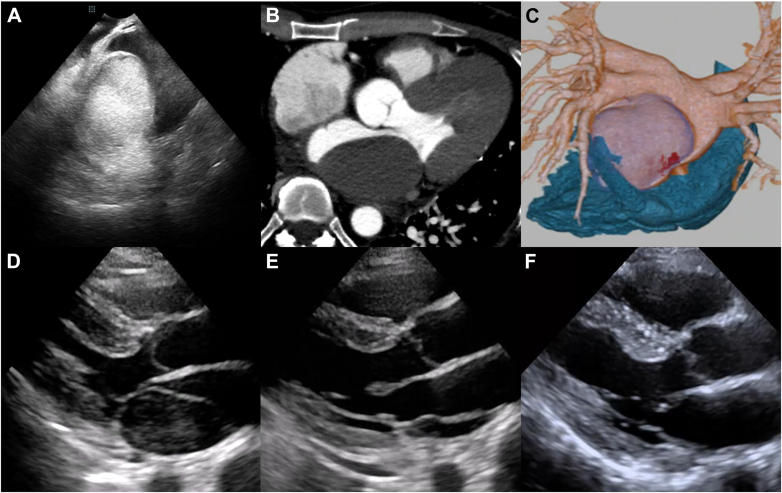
Multimodal Imaging and Temporal Change of Left Atrial Intramural Hematoma With Progressive Resolution (A) Intracardiac echocardiography imaging of LAIH. (B and C) Axial imaging of cardiac CT and 3-dimensional reconstruction of cardiac CT posterior view, showing the spatial relationship of the right heart, left atrium, pulmonary veins, and LAIH. (D to F) TTE images of the LAIH on postoperative day 1, postoperative day 4, and 4 months after discharge, respectively. CT = computed tomography; LAIH = left atrial intramural hematoma; TTE = transthoracic echocardiography.
